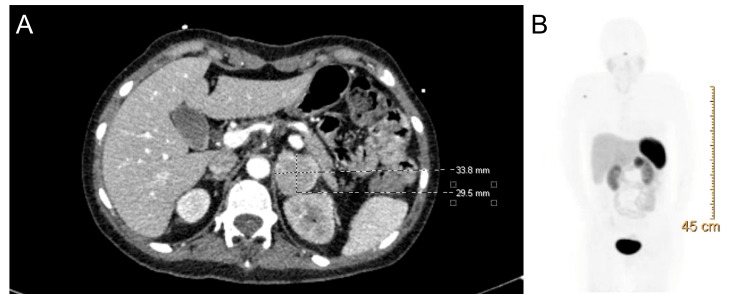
(A) CT abdomen and pelvis with IV contrast of left adrenal mass; (B) PET scan showing increased radiotracer uptake of mass superior to the left kidney. CT, computed tomography; PET, positron emission tomography; IV, intravenous
